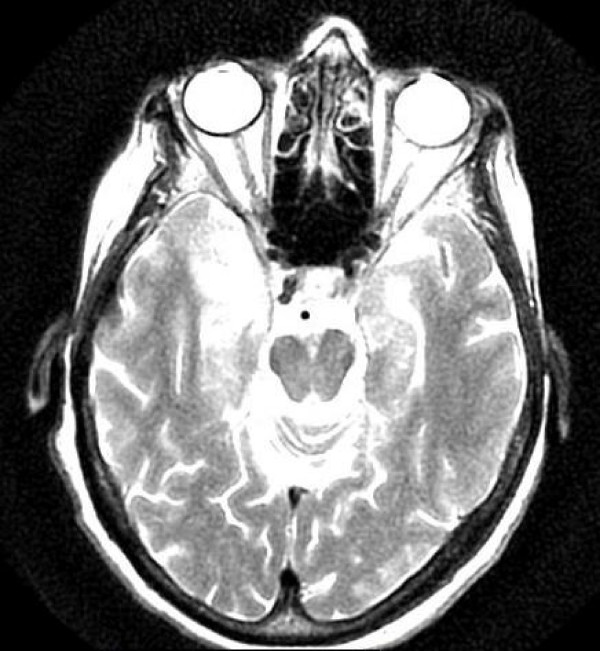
MRI showing abnormal signal intensity involving the medial aspect of the right temporal lobe.
